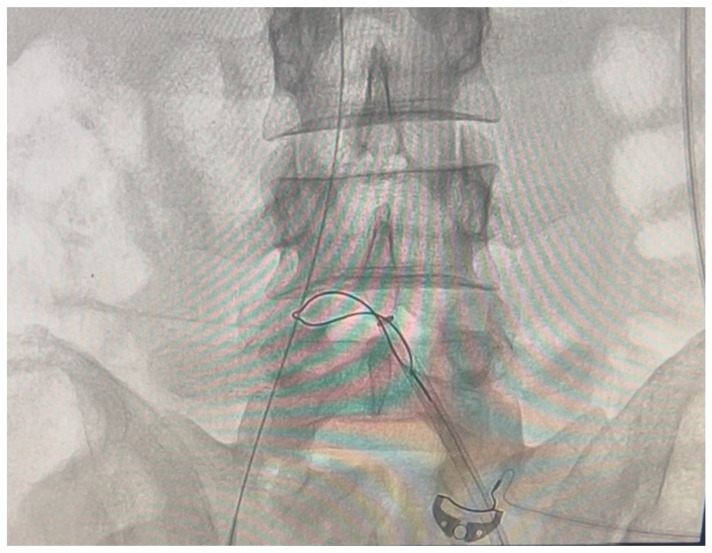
Radiography during the catheter removal with the Snare Kit.
